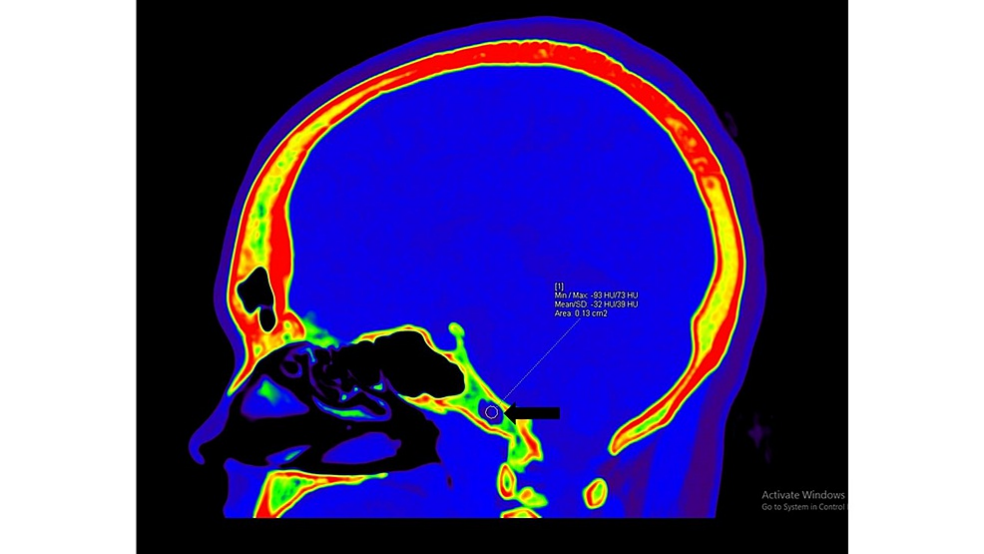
DECT image of the skull (sagittal section) demonstrating intraosseous lipoma at the clivus (black arrow) with ROI placement revealing fatty attenuation of the lesion. DECT, dual-energy computed tomography; ROI, region of interest
